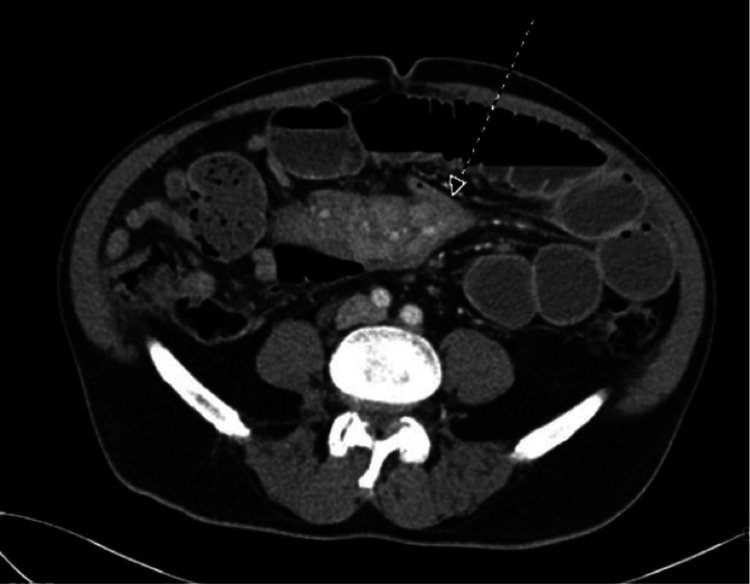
The axial image of the contrast-enhanced computed tomography scan of the abdomen and pelvis. The transverse section shows the transition point of the small bowel obstruction caused by the mesenteric tumor (arrow).
